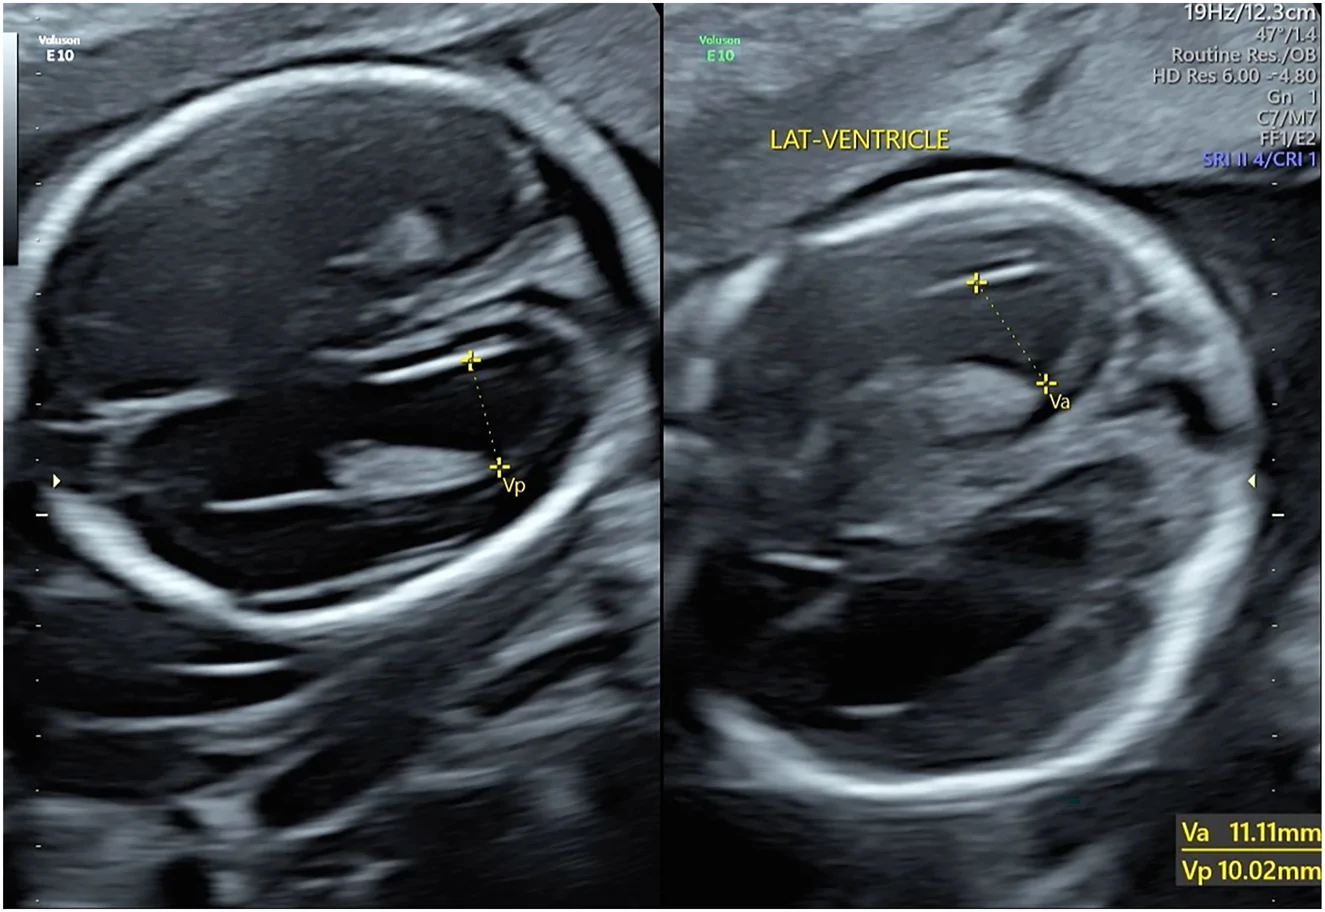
Ultrasound showing cerebral ventriculomegaly.
